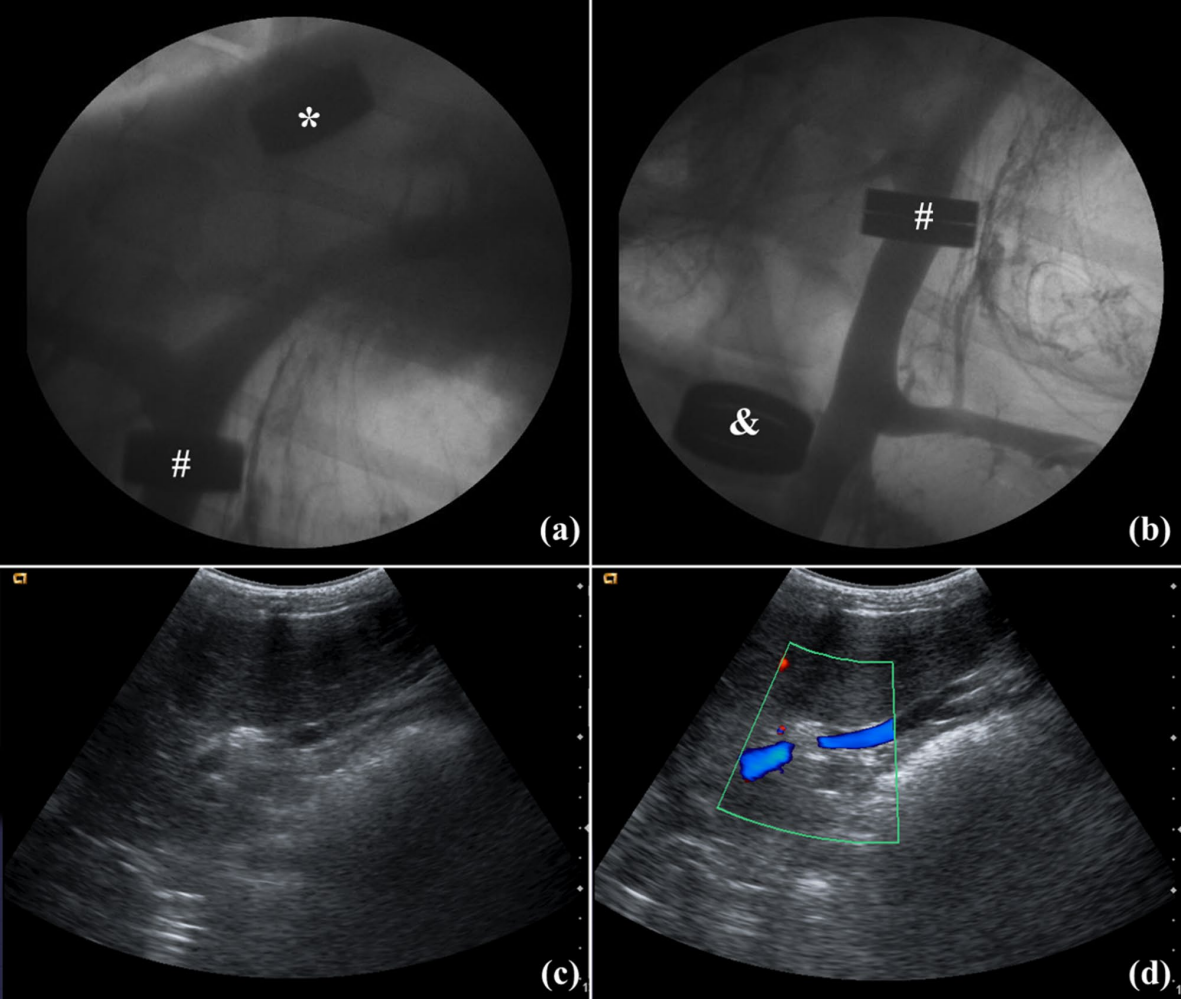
Postoperative anastomotic imaging examination. (a), (b) Postoperative portal venography: portal vein anastomotic stoma (#), suprahepatic (*) and infrahepatic inferior (#) vena cava anastomotic stoma; (c), (d) postoperative vascular anastomosis ultrasonography.
